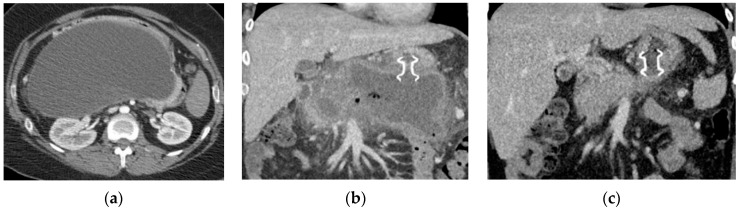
Computed tomography (CT) imaging of pancreatic pseudocyst. (a) Sagittal CT pancreas protocol image of a large 14.7 cm × 19.3 cm pseudocyst prior to lumen-apposing metal stent (LAMS) placement; (b) coronal CT image of pancreatic pseudocyst one day post-LAMS placement; and (c) coronal CT image of pancreatic pseudocyst six weeks post-LAMS placement.
